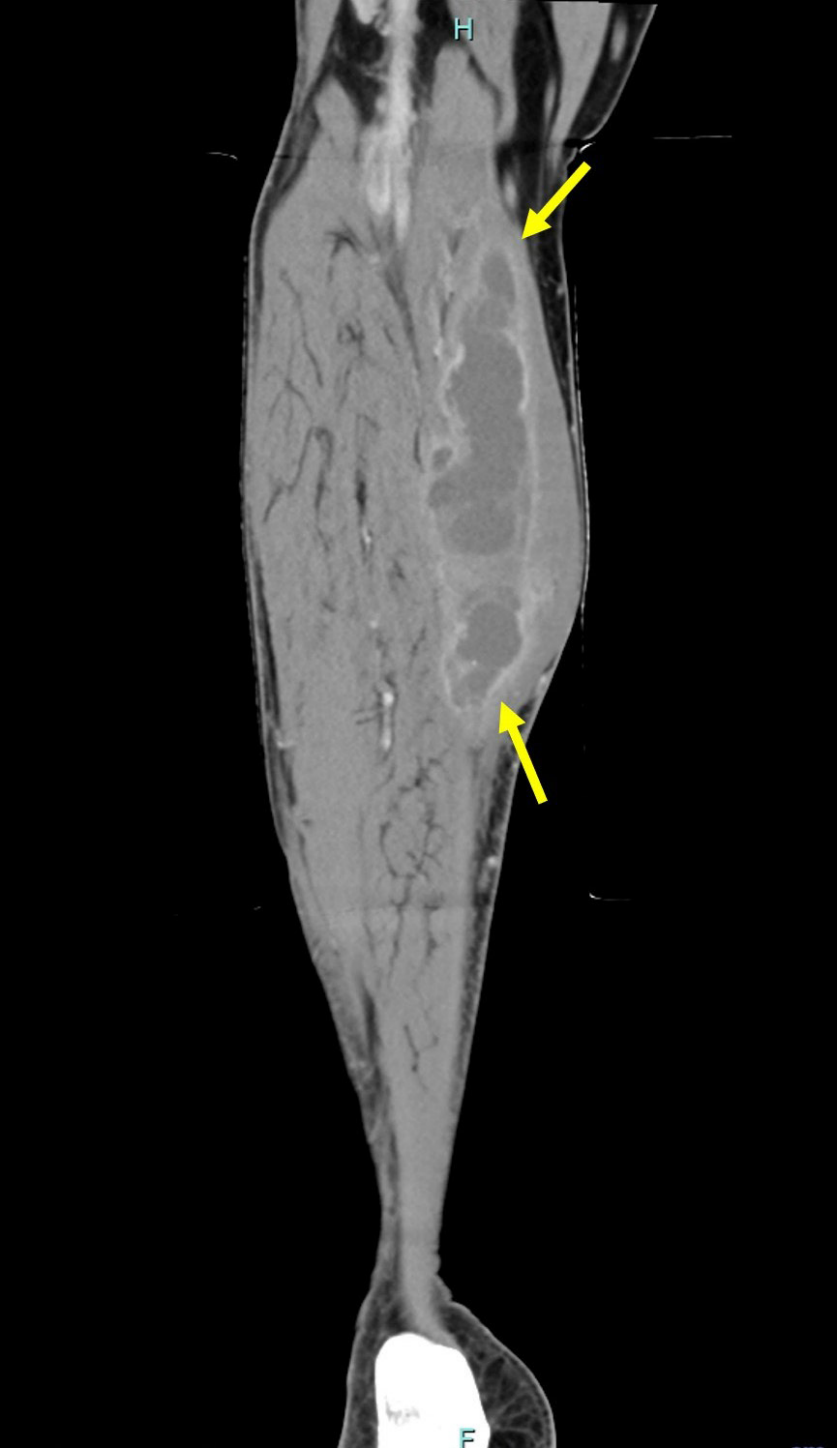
Contrast CT sagittal section of the right leg showing the extent of the multi‐loculated pseudotumor (arrows) within the calf.
